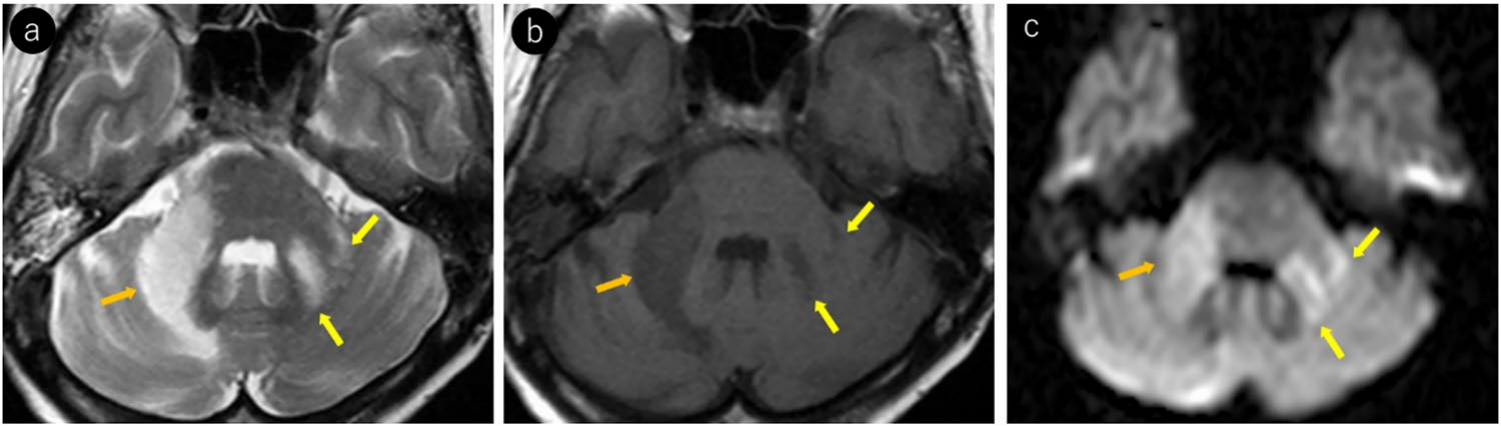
Woman in her 50 s with post-bone marrow transplantation for acute myeloid leukemia presented with progressive cerebellar ataxia. a T2-weighted imaging reveals hyperintense lesions around the right dentate nucleus extending to the right middle cerebellar peduncle, demonstrating a “shrimp sign” (orange arrows). Additional T2 hyperintense lesions were noted around the left dentate nucleus and left middle cerebellar peduncle (yellow arrows), showing an asymmetric distribution. b Lesions appear hypointense on T1-weighted images (orange and yellow arrows). c Most lesions demonstrate hyperintensity on diffusion-weighted imaging (orange and yellow arrows). The presence of JCV in the cerebrospinal fluid was confirmed and PML was diagnosed
